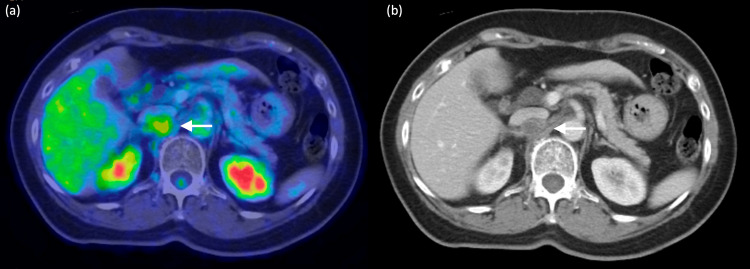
(a) FDG PET Scan. The retrocaval lesion showing FDG avidity (white arrow). (b) CT Scan: A retrocaval hypodense ovoid lesion (white arrow), demonstrating hypoenhancement on the portovenous phase.
